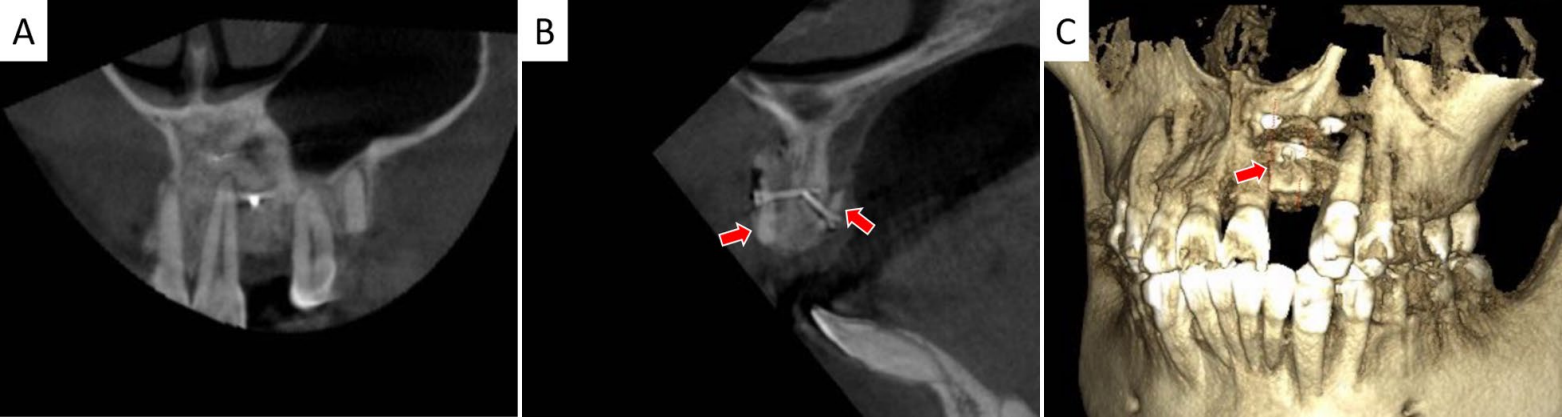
CBCT examination 4 months post-operation. A–C showed coronal-, sagittal plane and 3D reconstruction views. Red arrows depict TS integrated with host bone
